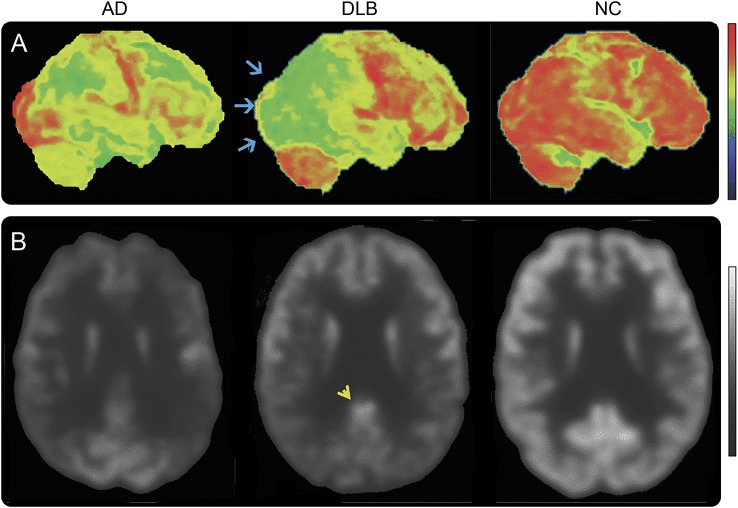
^18^F-FDG-PET images in Alzheimer disease (AD), dementia with Lewy bodies (DLB), and normal controls (NC) (A) Right lateral metabolic surface map projection. (B) Standard axial view transecting the posterior cingulate region. Occipital lobe metabolism is preserved in AD and NC but reduced (blue arrows) in DLB. Hypometabolism in AD is predominantly in the temporal, parietal, and frontal regions. There is normal metabolism as reflected by the normal ^18^F-FDG uptake (lighter shade of gray) in the posterior cingulate region (yellow arrowhead) surrounded by reduced ^18^F-FDG uptake (darker gray) in the adjacent occipital cortex in DLB, representing the cingulate island sign. This contrasts with the relatively reduced ^18^F-FDG uptake in the posterior cingulate and relatively preserved ^18^F-FDG uptake in the occipital cortex regions in AD. In the control, there is normal ^18^F-FDG uptake in the posterior cingulate, occipital, and other neocortical regions. Color and grayscale sidebars show increasing degrees of deviation from normal as the signal trends lower in the sidebars (red is normal while black is maximally abnormal in color images; white is normal while black is maximally abnormal in grayscale images). Reproduced with permission from Dr. Val Lowe, Mayo Clinic, Rochester, MN.
